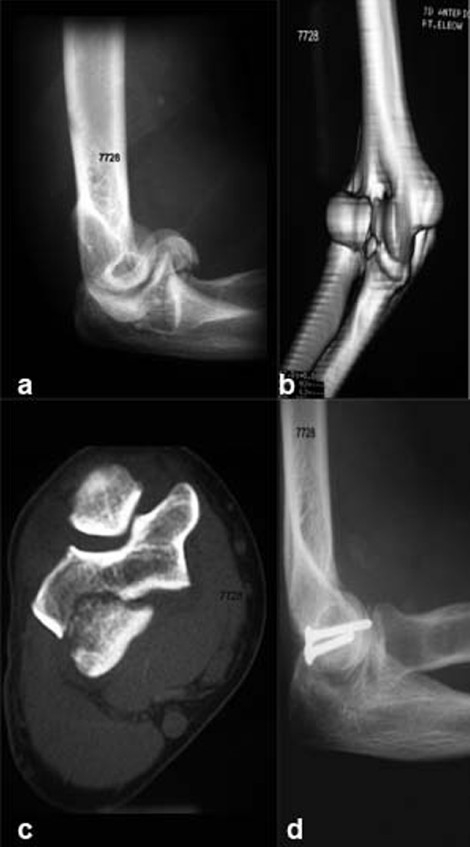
(a) X-ray showing double arc sign, fragments displaced distally. (b) 3D CT scan showing the involvement of the lateral trochlear ridge. (c) Axial section of CT scan showing the lateral trochlear ridge fracture. (d) Lateral X-ray of the elbow showing fixation with 2.7 mm screws
